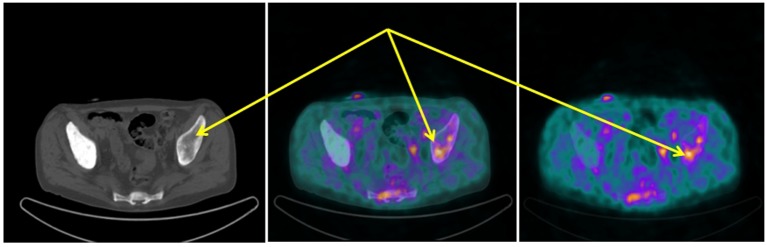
Same patient and acquisition parameters of Fig. 1. ^68^Ga-DOTANOC transaxial PET/CT (left CT attenuation correction, middle fused PET/CT, right PET only) showing increased uptake corresponding to lymphangitic carcinomatosis of the right lung (arrows).
